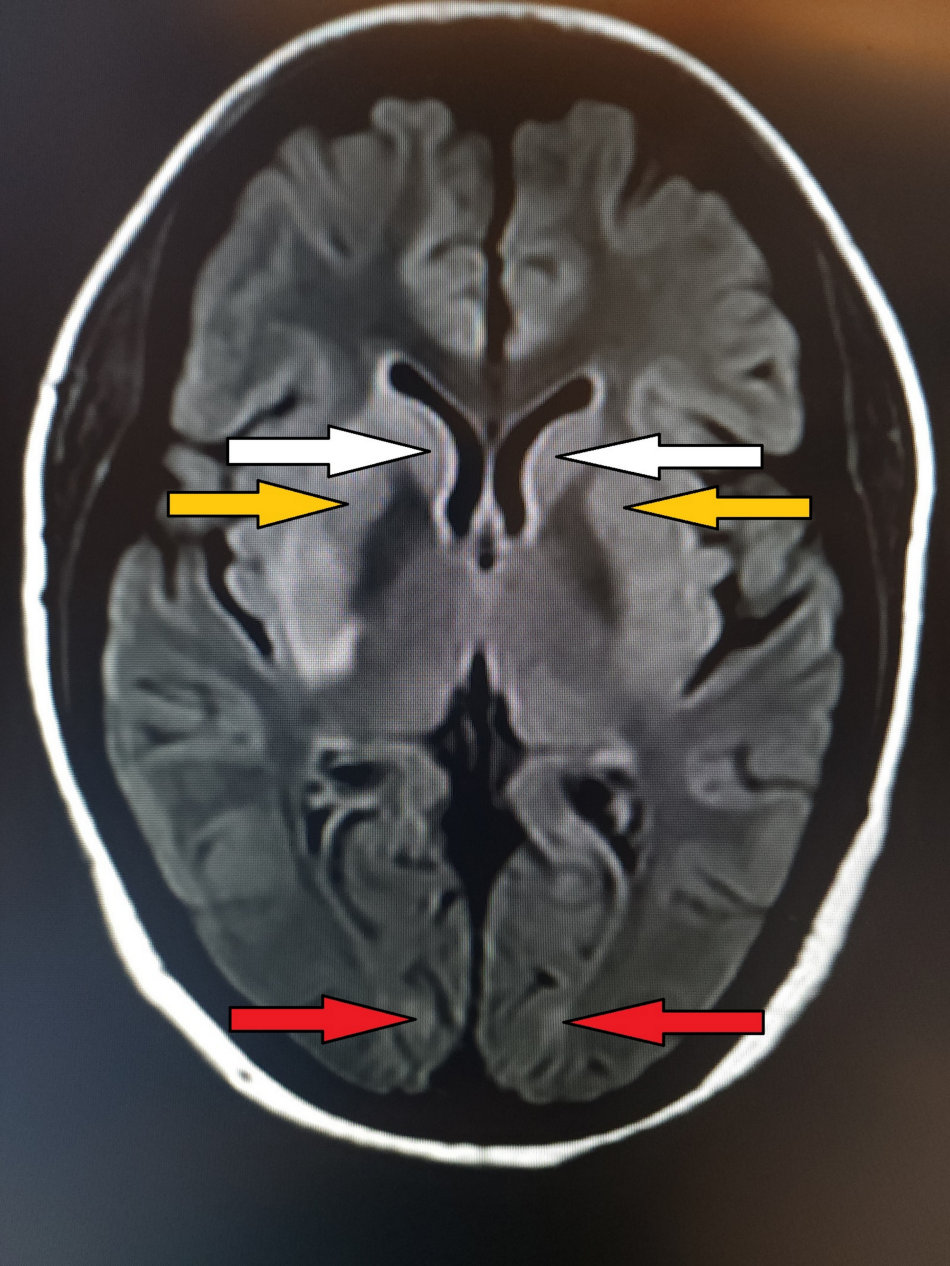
MRI brain (FLAIR) showing bilateral hyperintense lesions at multiple sites. White arrows show hyperintensities in the head of the caudate nucleus bilaterally; Yellow arrows show hyperintensities in the putamen region bilaterally; Red arrows show hyperintensities in the occipital region bilaterally. MRI: magnetic resonance imaging; FLAIR: fluid-attenuated inversion recovery
